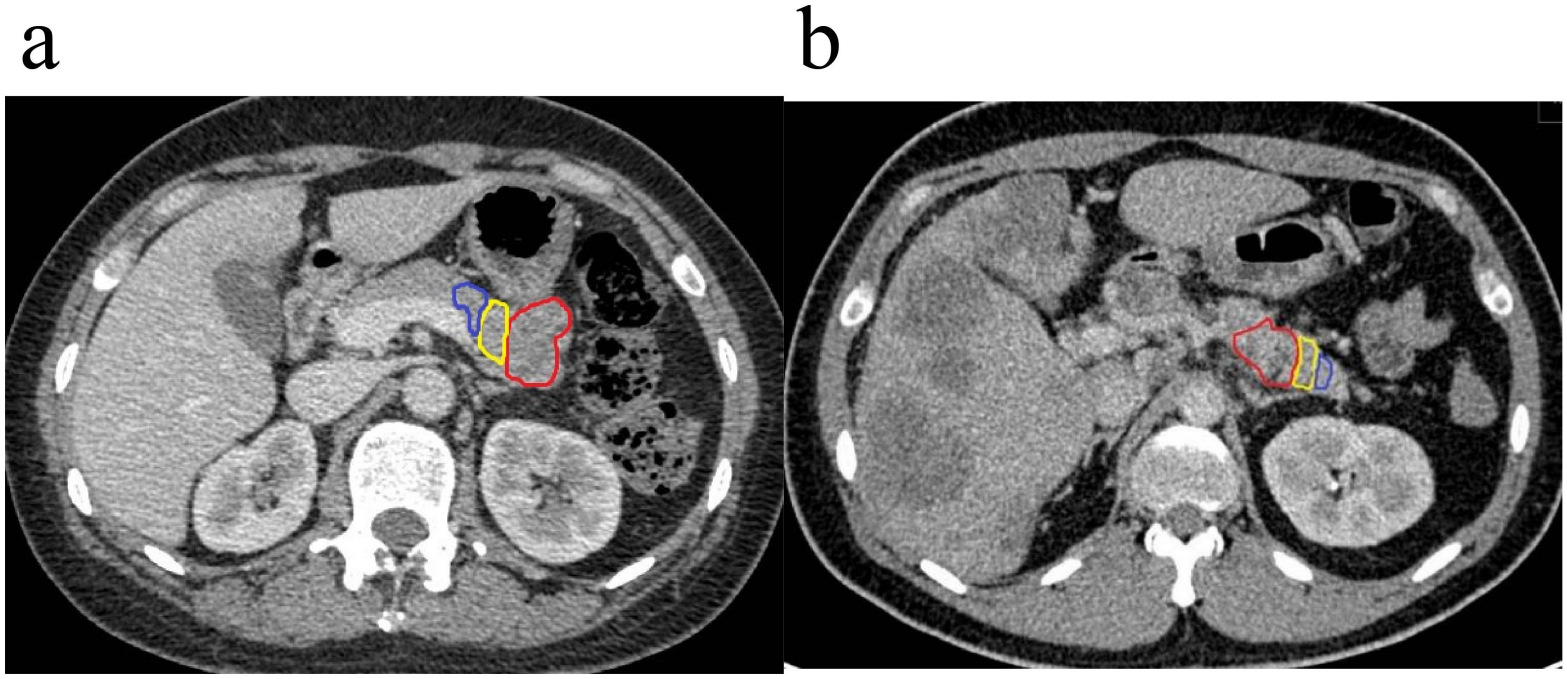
Region of interest (ROI) delineated in axial portal phase computed tomography (CT). (a) A 63-year-old male patient was diagnosed with G3 pancreatic neuroendocrine tumor (pNET) at the tail of the pancreas, and the space between the adjacent gastric body fat had disappeared. (b) A 34-year-old male patient presented with pancreatic neuroendocrine carcinoma (pNEC) in the pancreatic body, with the tumor invading the nearby splenic artery, showing rough and localized narrowing, multiple intrahepatic metastases, and peritoneum multiple enlarged lymph nodes (the red area is the tumor body, the yellow area is peritumor (a) the blue area is peritumor (b).
